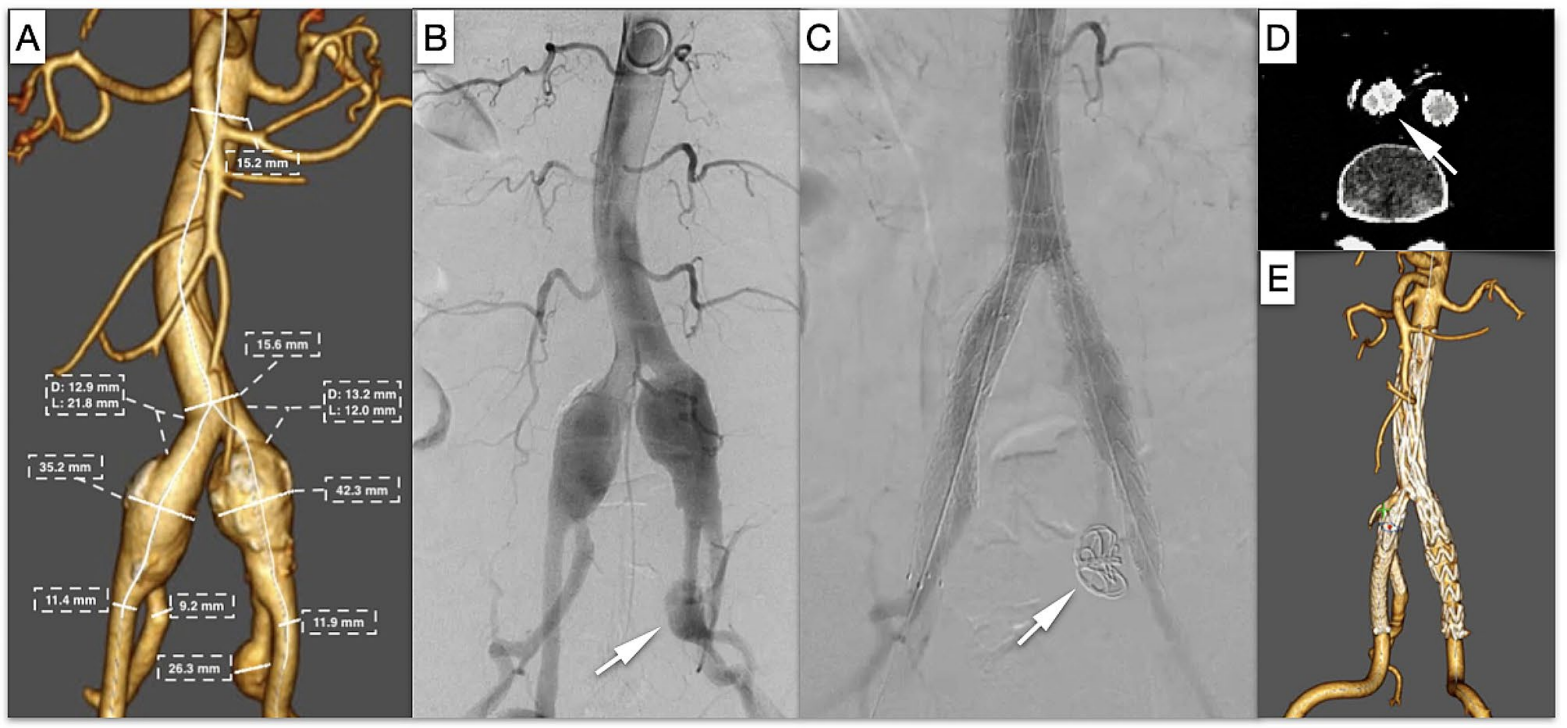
Case 3: A Preoperative CT imaging and measurement of aneurysm anatomical parameters. The diameter of the right proximal CIA was 12.9 mm, the diameter of the left proximal CIA was 13.2 mm, and the length of the nonaneurysmal proximal CIA was 12.0 mm. B Intraoperative angiogram. The left IIA was aneurysmal (white arrow), measuring 26.3 mm in diameter. C The final angiogram demonstrated successful deployment of Aegis™ and two parallel covered stents in the right iliac artery. The left IIA aneurysm was embolized by coils (white arrow). E The cross-sectional image of the two-year follow-up CT scan demonstrated the “eye of the tiger” configuration (white arrow), with no evidence of gutter leakage. F CT scan reconstruction in three dimensions
